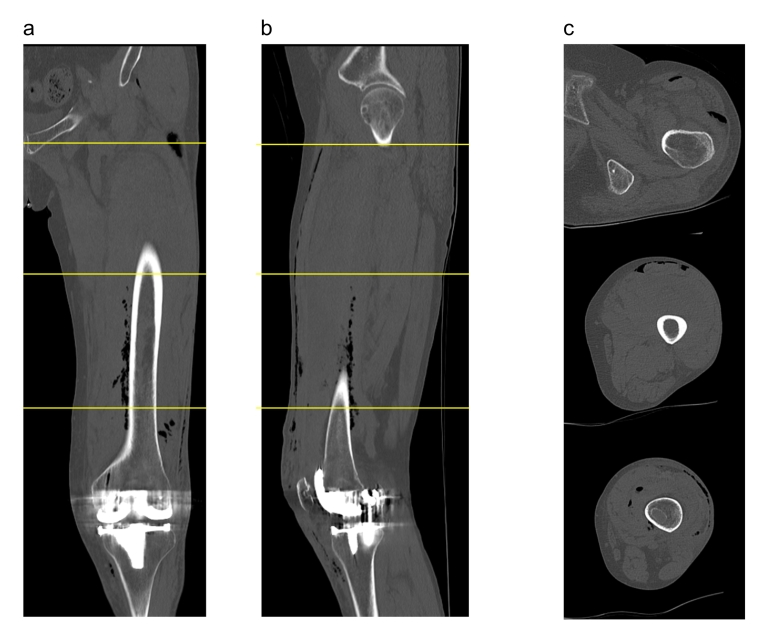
A CT scan was performed on the second day after the operation. a) The coronal image reveals the presence of emphysema extending to the hip joint. b) The sagittal image displays emphysema along the quadriceps fascia and around the femur. c) Three axial images, from proximal to distal, demonstrate extensive spread of emphysema.
